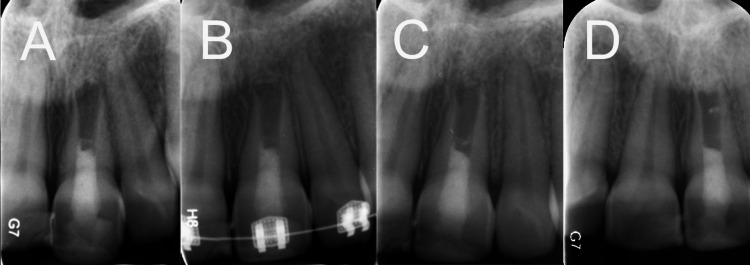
Radiographic follow-ups of the REP-treated tooth undergoing orthodontic treatment (A) A two-year follow-up radiograph revealing symptom resolution and signs of bony healing under the calcium-enriched mixture (CEM) cement, although root development remained limited. (B) Three years post-REPs, orthodontic intervention was introduced, with the specifics detailed in the report. (C) A follow-up radiograph one year after the initiation of orthodontic treatment, demonstrating a discernible increase in the root length and thickness. (D) A three-year follow-up radiograph indicating a normal periodontal ligament, a slight improvement in the root wall thickness and length. REP, regenerative endodontic procedure
